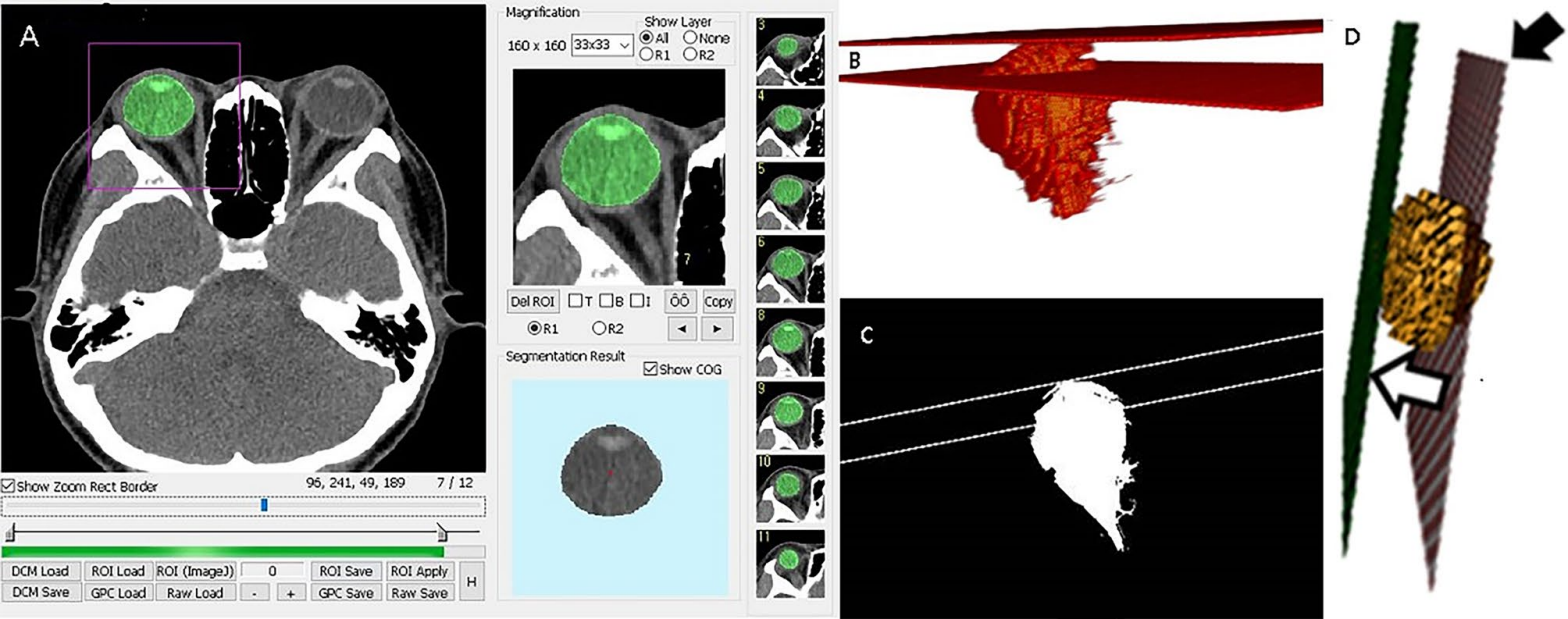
Example images were produced using our 3D reconstruction software. (A) Recognizing the eyeball of the whole axial Section (60–70 section) of computed tomography using automatic segmentation. (B) 3D rendering images of the interzygomatic plane and the plane of eyeball surface from a normal vector on the segmented eyeball (C) Binary image for mathematical verification (D) 3D reconstruction of the eyeball (yellow sphere) and the interzygomatic plane (black arrow). A perpendicular line (white arrow) drawn from the recognized eyeball surface to the interzygomatic plane by 3D reconstruction software.
